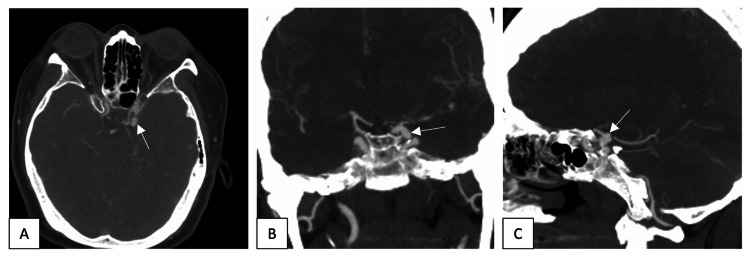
Axial (A), coronal (B), and sagittal (C) CT angiograms showed a left supraclinoid internal carotid artery multi-lobular aneurysm
